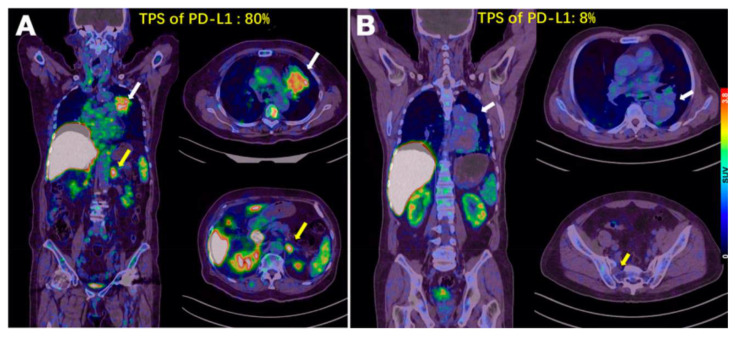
(A) An 80-year-old woman with advanced NSCLC and a PD-L1 tumor proportion score (TPS) of 80%. The SUVmax of the primary tumor was 4.87 (white arrow), and that of the left adrenal metastasis was 5.47 (yellow arrow) on [^68^Ga]Ga-NOTA-WL12 PET. (B) A 68-year-old man with a PD-L1 TPS of 8%. The SUVmax of the primary tumor in the left lung (white arrow) and right sacral metastasis (yellow arrow) was 1.84 and 0.8, respectively, on [^68^Ga]Ga-NOTA-WL12 PET. Reprinted/adapted with permission from Ref. [96]. 2021 by the Society of Nuclear Medicine and Molecular Imaging, Inc.
